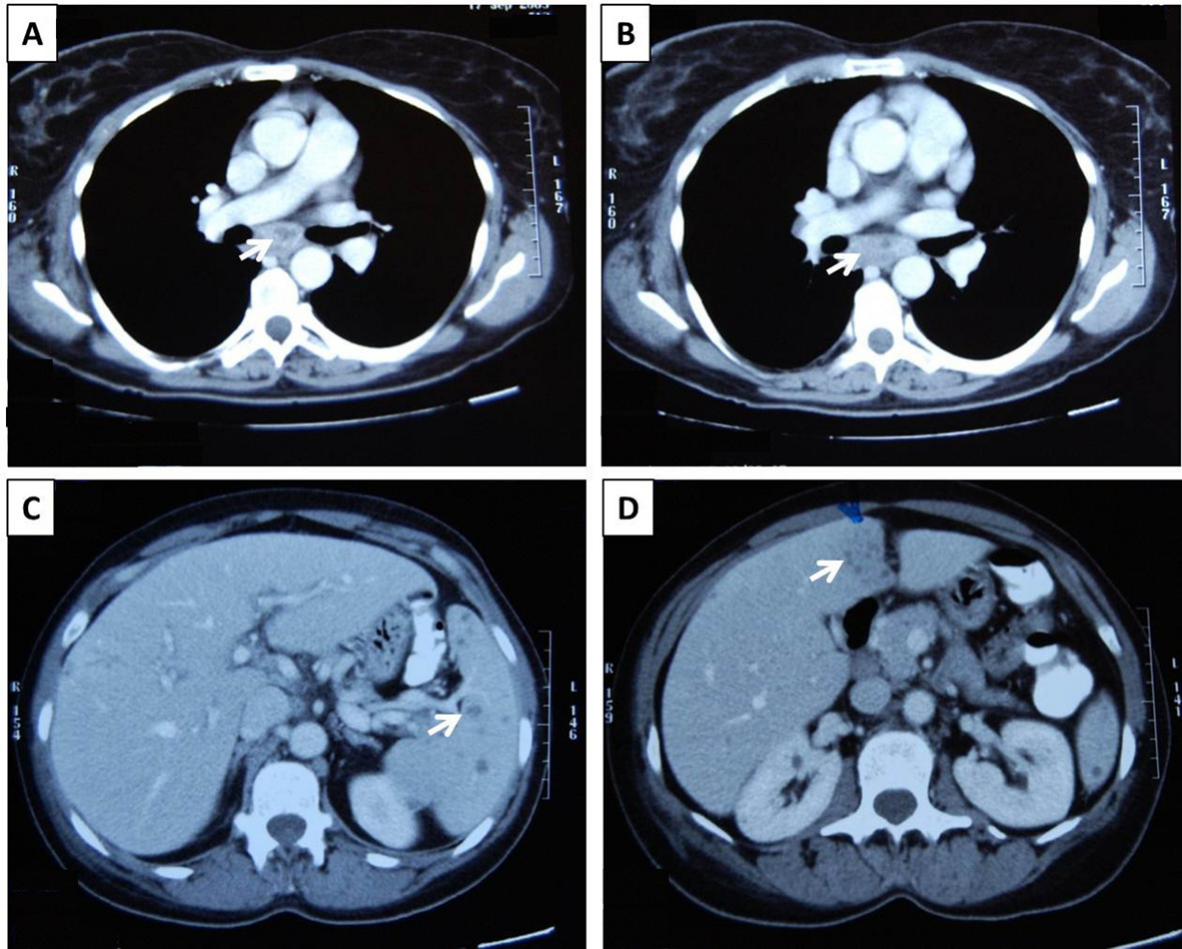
Thoracic and abdominal CT scans from the presented patient: Enlarged mediastinal lymph nodes with a hypodense center (A, B, white arrows), and multiple hypodense splenic (C, white arrow) and hepatic (D, white arrow) lesions were detected.
